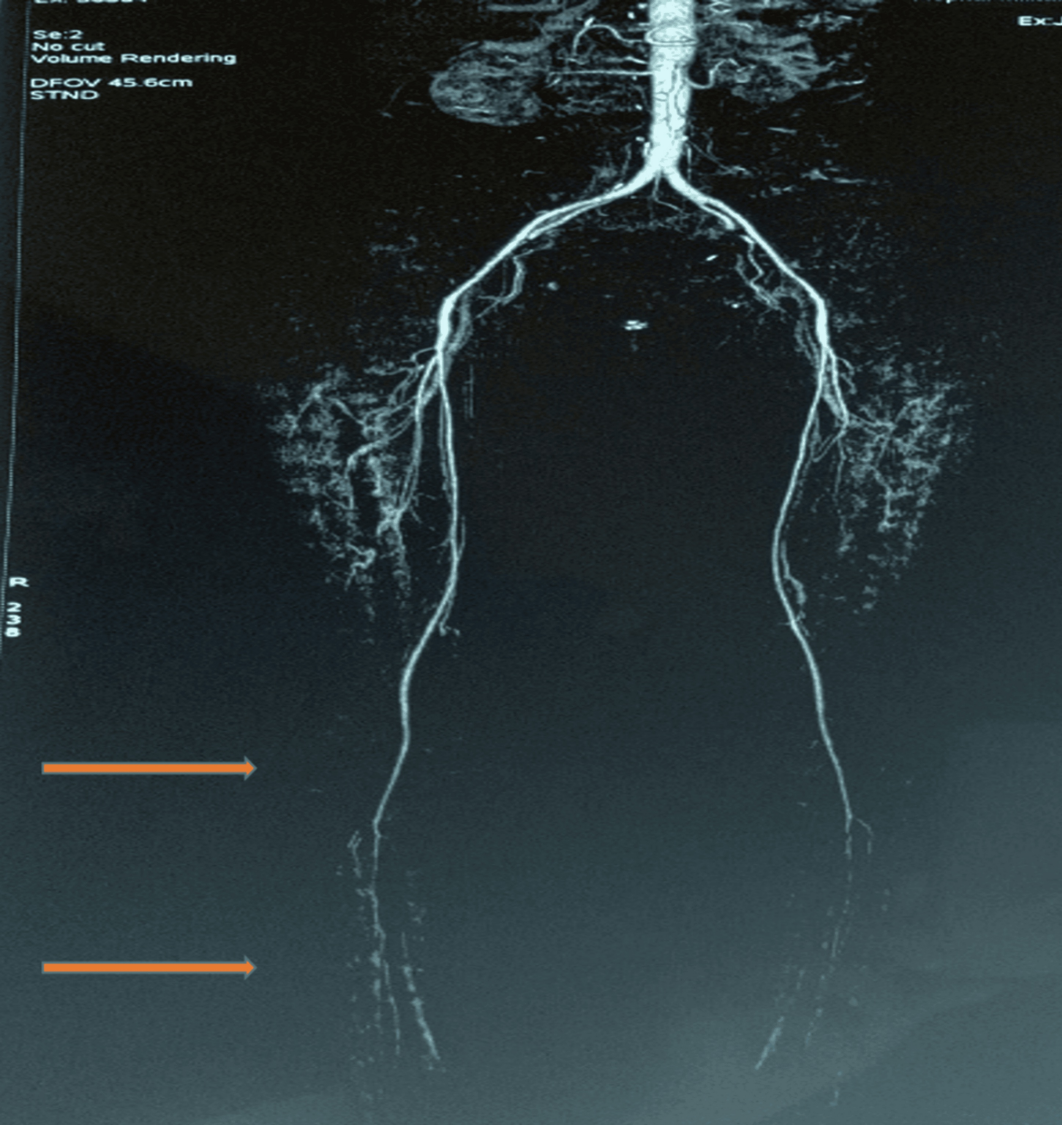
CT angiography of both lower limbs showing diffusely small-caliber arteries with long, filiform (thread-like) stenoses.
